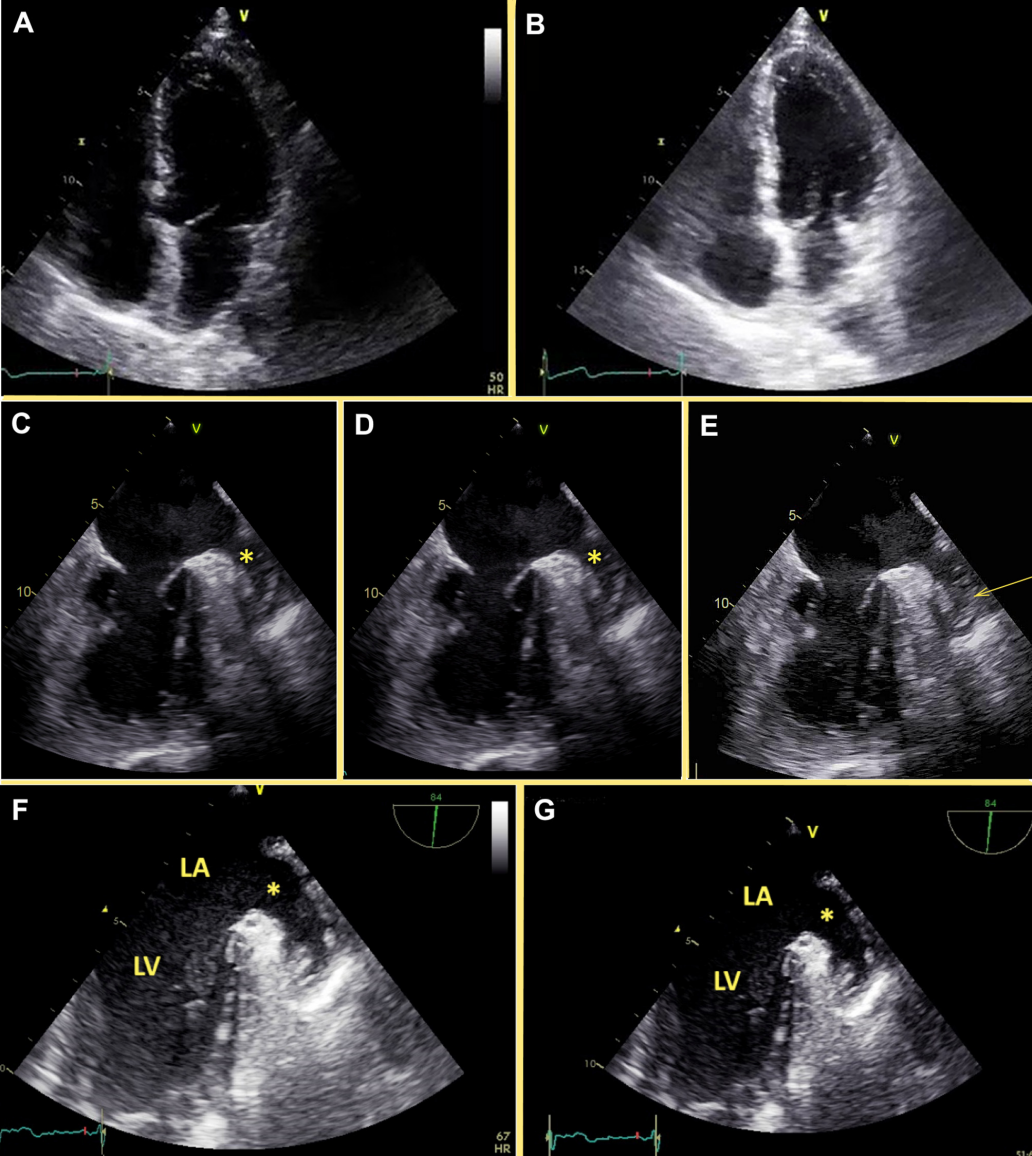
Effect of different frame rates and compression on the echocardiography image quality. (A,B) Apical 4-chamber view of the same patients recorded at two different frame rates. (A) Left – at frame rate 44 fps, (B) Right – at frame rate 74 fps. Image with a higher frame (B) rate contains more echocardiography information than the image with a lower frame rate (A). (C–E) TEE of the patient with atrial fibrillation before cardioversion. (C) Raw data. Swirling is present in the left atrium and the appendage (starr). (D) RLE lossless compression. Swirling is present in the left atrium and the appendage (starr). (E) Lossy compression. Significant swirling in the left atrium and is suspicious for thrombus in the left atrial appendage (arrow). (F,G) TEE of the patient with atrial fibrillation. (F) raw data, there is some swirling in the left atrium. (G) lossy compression, there is no swirling at all. LV, left ventricle; LA, left atrium.
